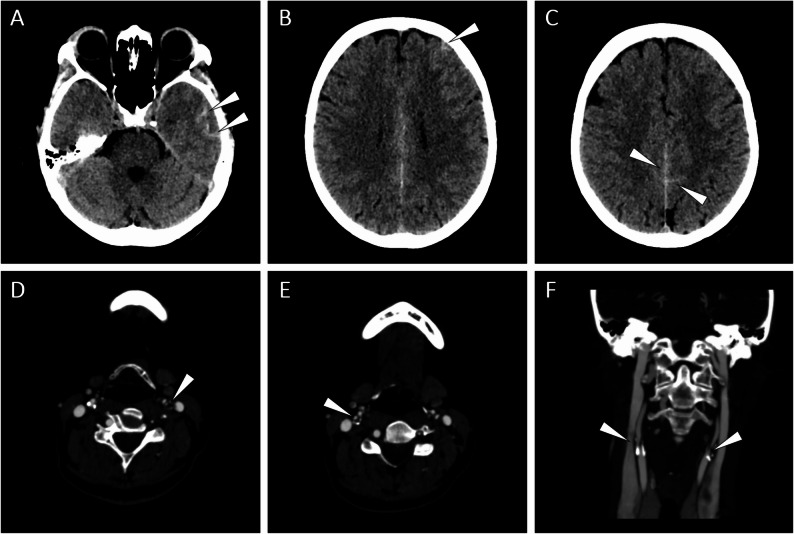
Acute cranial/carotid CT/CTA imaging. Axial non-contrast CT scans showing left temporal (A), left frontal (B), and left parafalcine cSAHs (C). Axial carotid CTA demonstrating subtotal occlusion of the ipsilateral ICA (D) and a significant stenosis of the contralateral ICA (E). Coronal CTA showing bilateral atherosclerotic plaques of the stenotic ICAs (F). cSAH, convexity subarachnoid haemorrhage; CT, computed tomography; CTA, CT angiography; ICA, internal carotid artery
